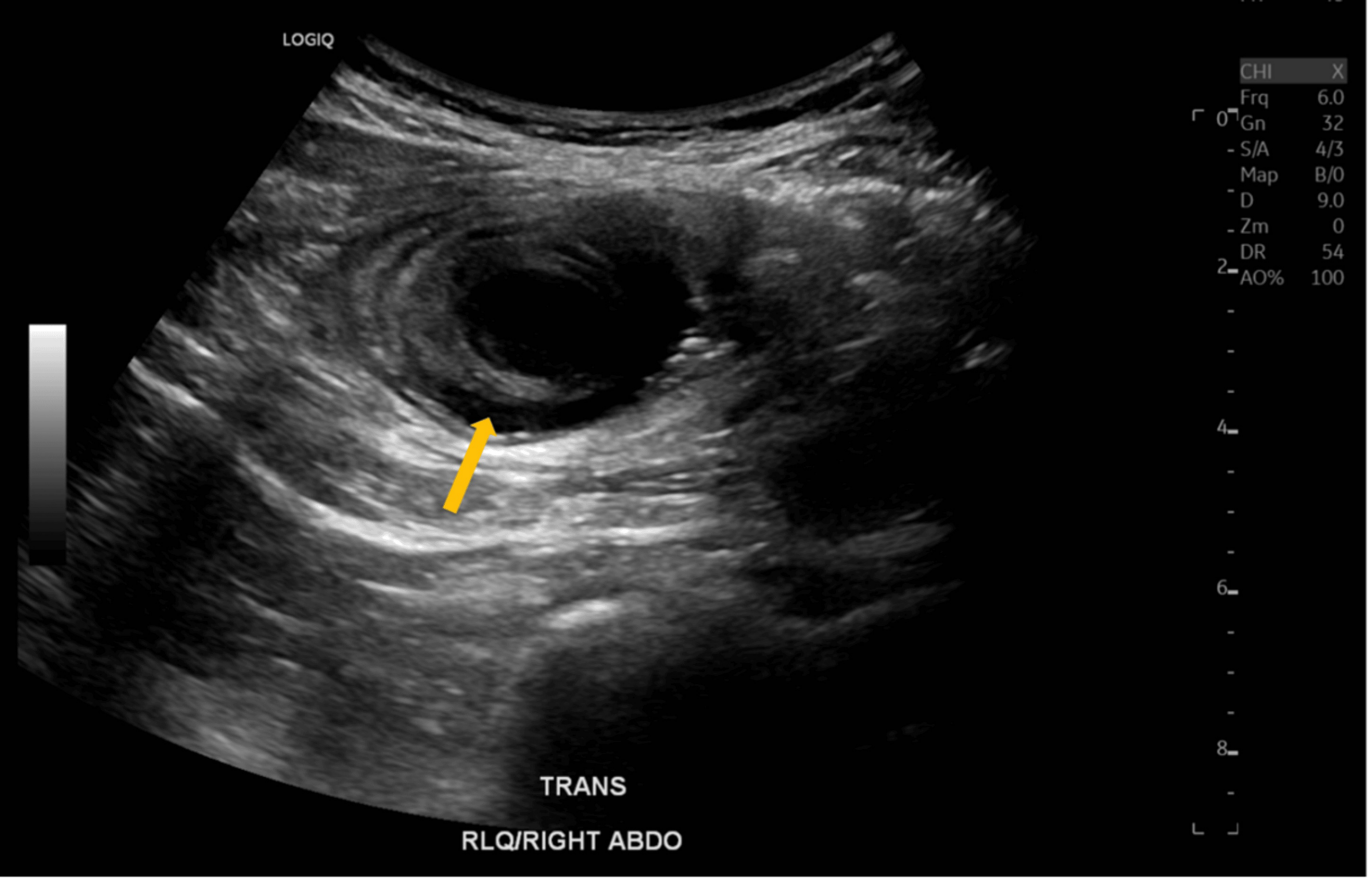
Initial abdominal ultrasound on presentation An arrow indicates intestinal intussusception in the right lower quadrant.
